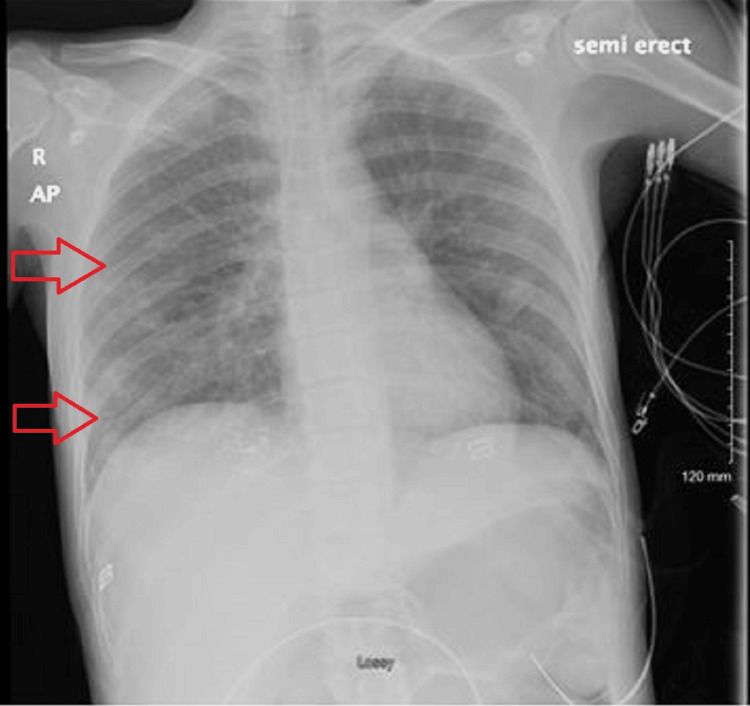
Chest X-ray showing extensive septic emboli and consolidative changes.
